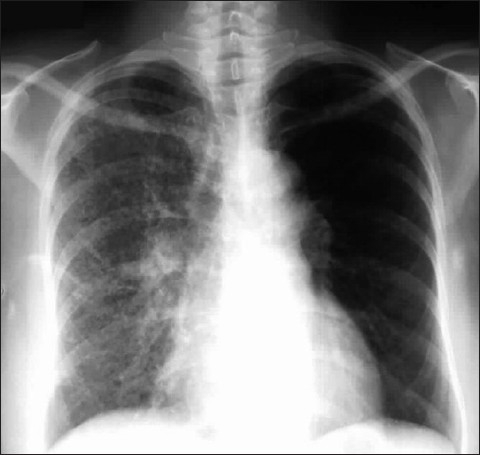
Supine portable chest radiograph showing extensive air space shadowing throughout the whole of the right lung and the left lung base due to alveolar pulmonary edema with associated pleural effusions secondary to heart failure. Note the air bronchograms in the right upper zone, sometimes seen with congestive heart failure
